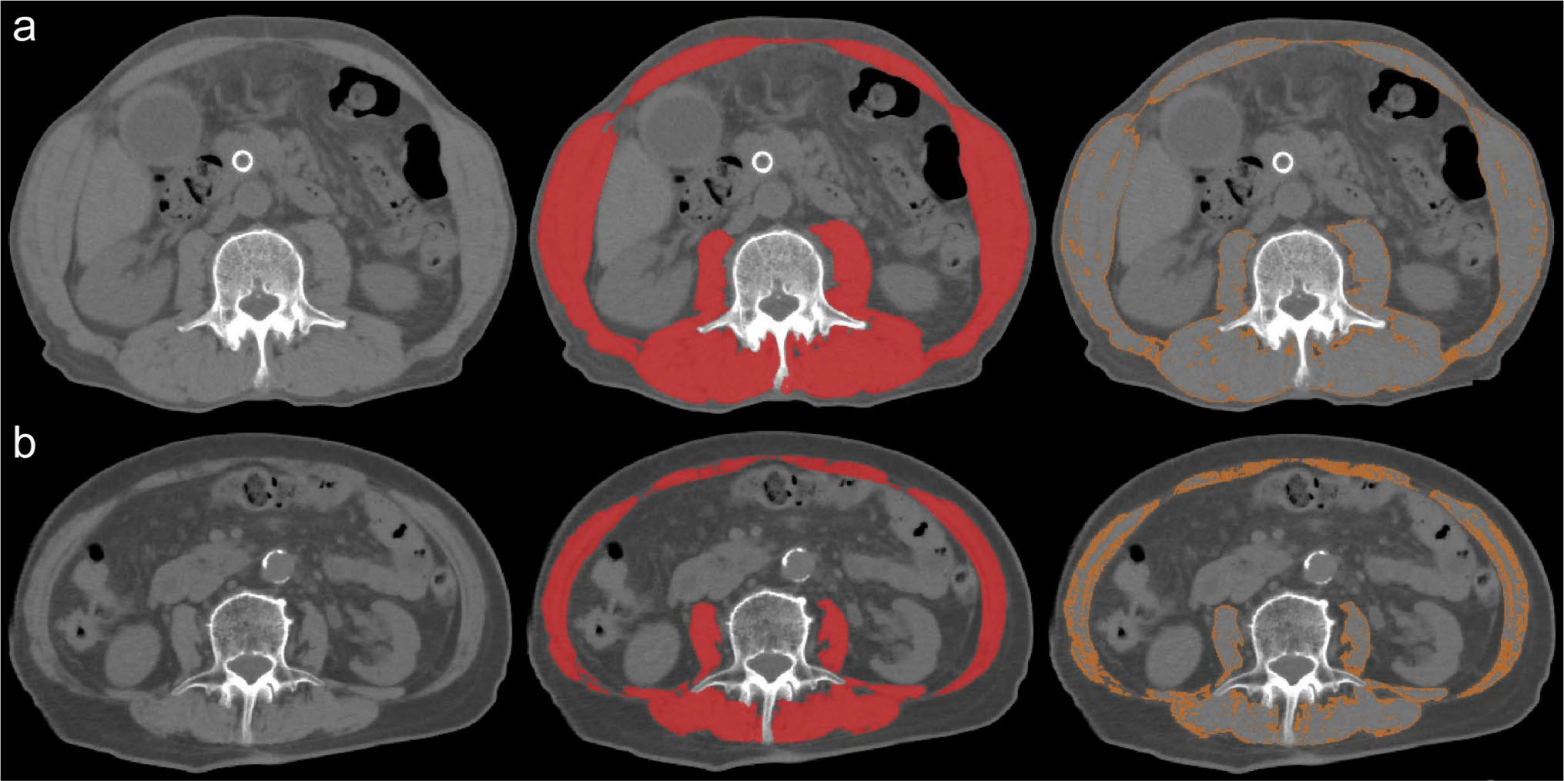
Computed tomography-based assessments of volume and density of skeletal muscles at the level of the third lumbar vertebra [left, non-annotated non-enhanced image; middle, annotated with skeletal muscle area (− 29 to + 150 HU); right, annotated with relatively low-density areas of skeletal muscle (− 29 to + 29 HU) suggesting lipid infiltration]. a Case with extrahepatic bile duct cancer showing high levels of skeletal muscle volume and density. b Case with extrahepatic bile duct cancer showing low levels of skeletal muscle volume and density. Of note, body mass indexes of the two patients were at the same level (a, 20.9 kg/m^2^ and b, 21.7 kg/m^2^). *HU* Hounsfield unit
